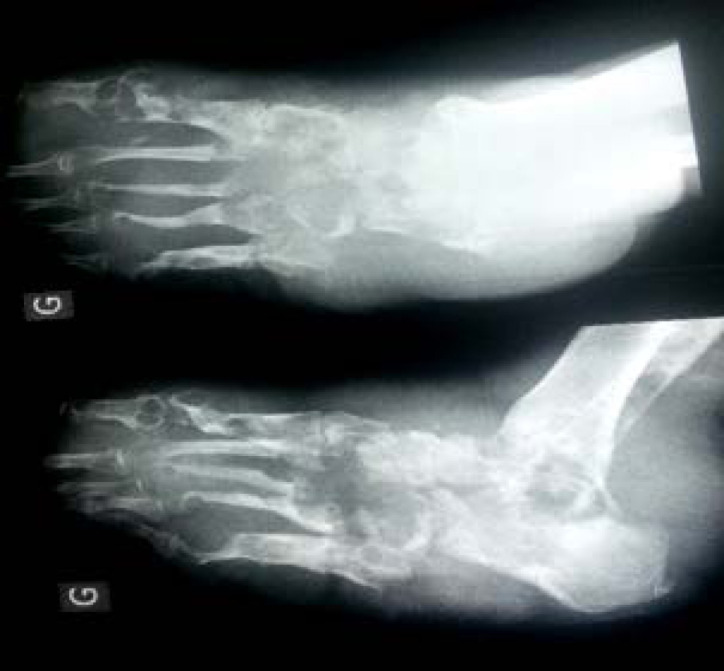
Radiographie objectivant une atteinte ostéoarticulaire avec lyse osseuse d'un eumycétome pied gauche évoluant depuis 20 ans chez un homme, 62 ans, cultivateur X-ray showing osteoarticular involvement with bone lysis of a left foot eumycetoma evolving for 20 years in a 62-year-old male farmer
